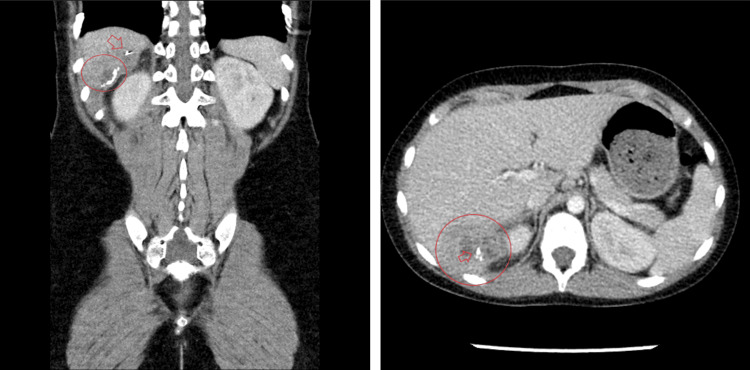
Computed tomography images showing a hepatic abscess taken one day before admission. The coronal and axial views shown display clearly the lesion in question (circled region), with early evidence of calcifications (radiolucent areas marked with arrows). In the coronal view, an area of aberrant calcification is marked. The red circle shown on the axial view shows inflammation of the kidney and liver; the heterogeneous lesion is clearly noted in the liver, and locally extends medially to the kidney.
